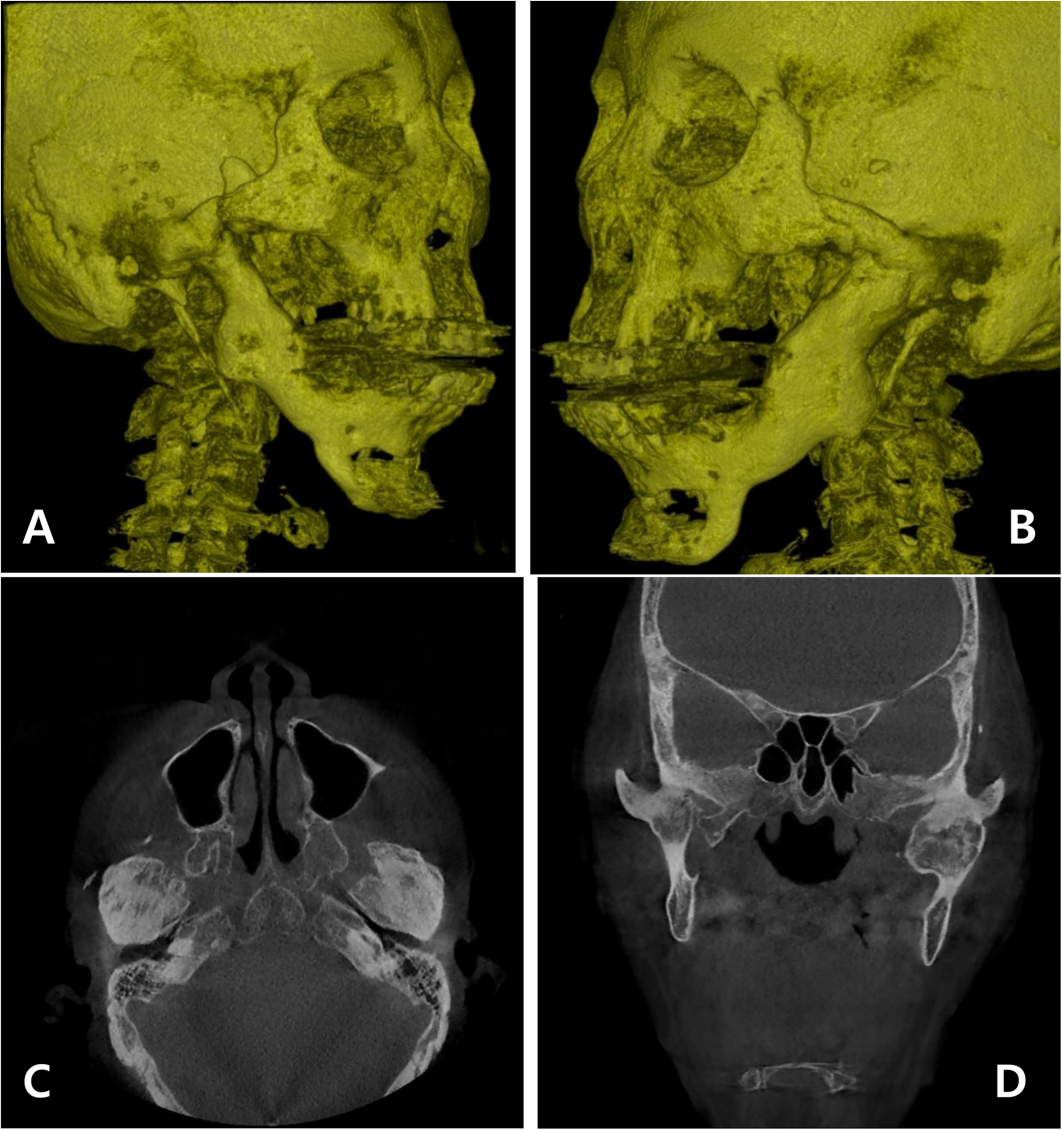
The computed tomogram (CT) images taken before total joint reconstruction surgery. Complete bony ankylosis of the temporomandibular joints (TMJs) at both sides was observed in the three-dimensional (3D) reorientation views (a, b), axial view (c), and coronal view (d)
